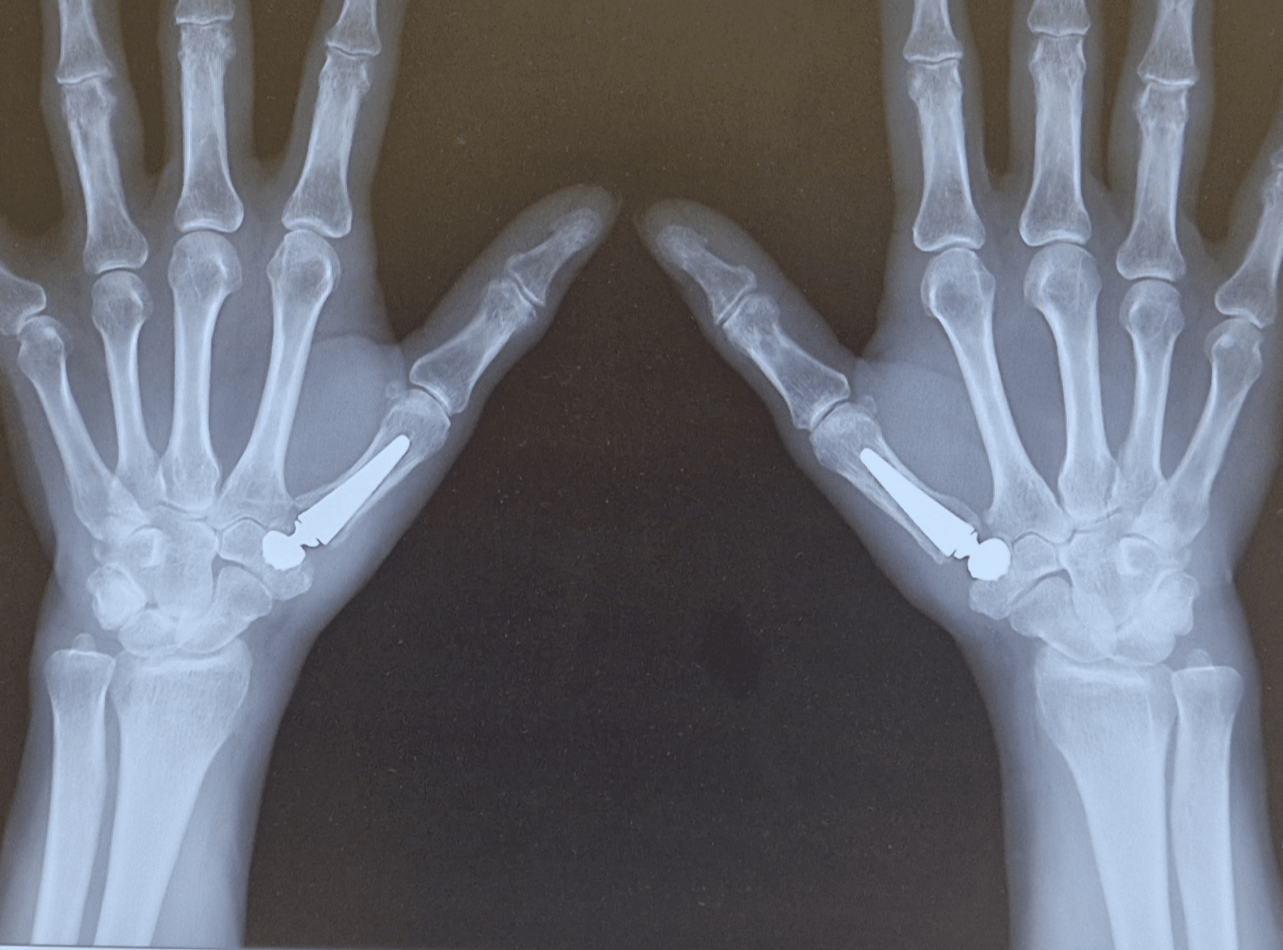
Postoperative anteroposterior (AP) X-ray
